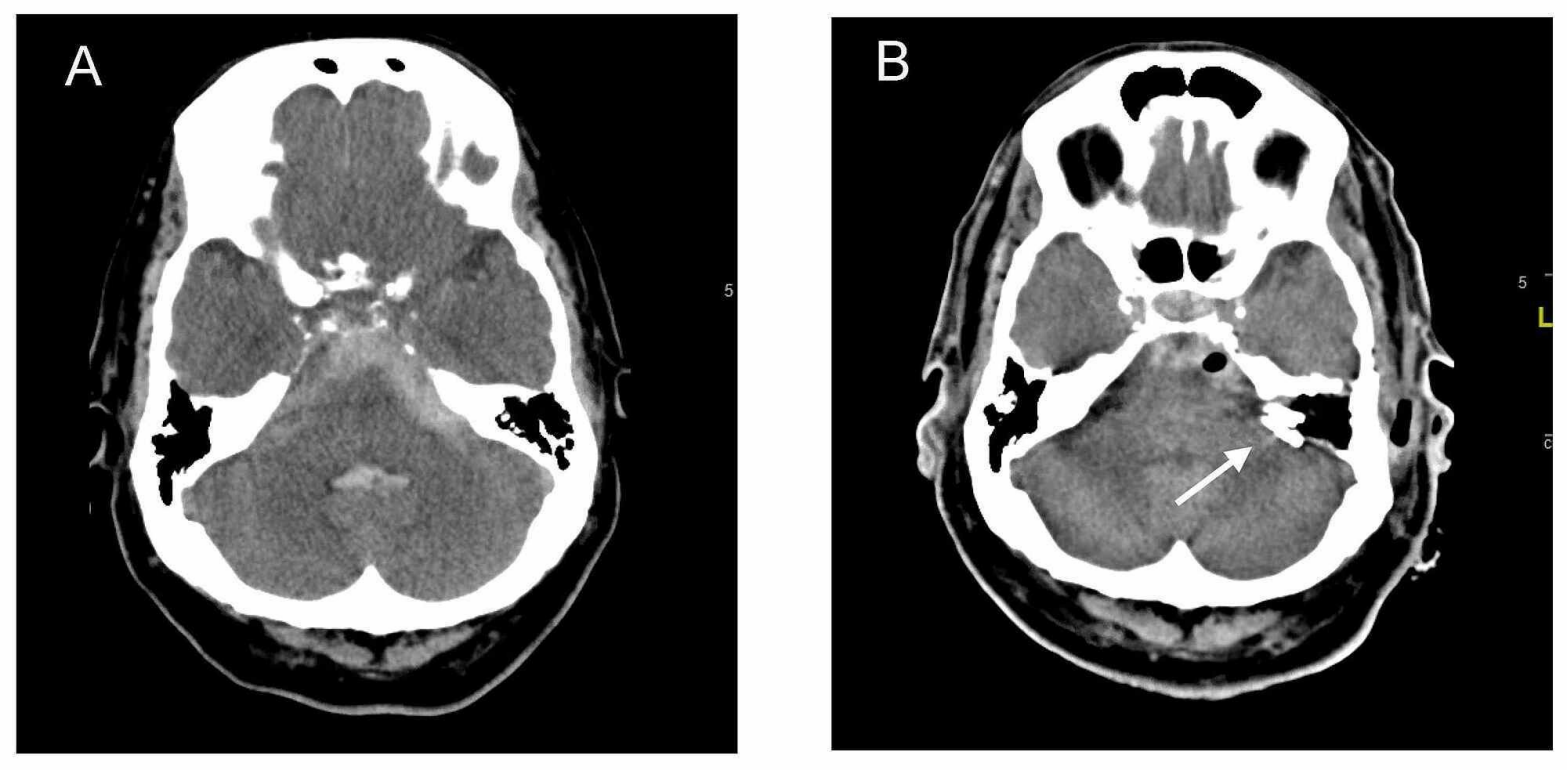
Pre- and post-op CT head Admission CT head without contrast revealed a Fisher grade 4 SAH with extension of hemorrhage into the fourth ventricle. The SAH pattern is more localized at the left cerebellopontine angle cistern, close to the internal auditory canal (A). Post-operative CT head revealed the location of the clips near the left IAC (B). CT, Computed topography; IAC, internal auditory canal; SAH, subarachnoid hemorrhage.
